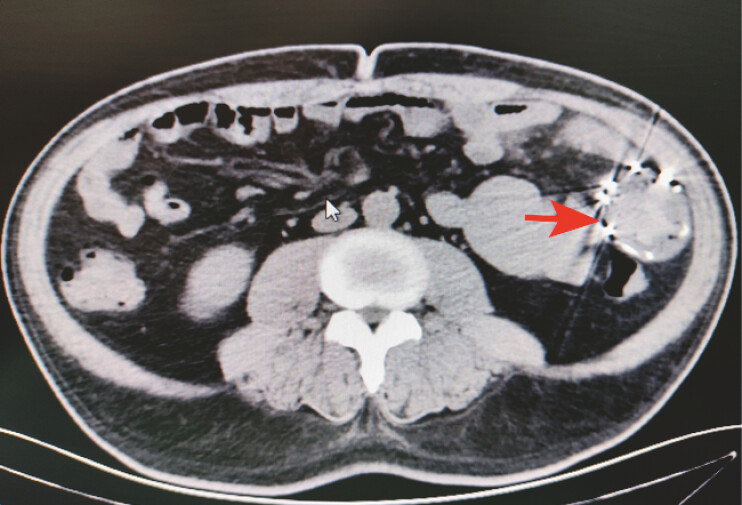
A computed tomographic image showing an metallic stent-like high-density shadow in the left mid-abdomen (red arrow).
